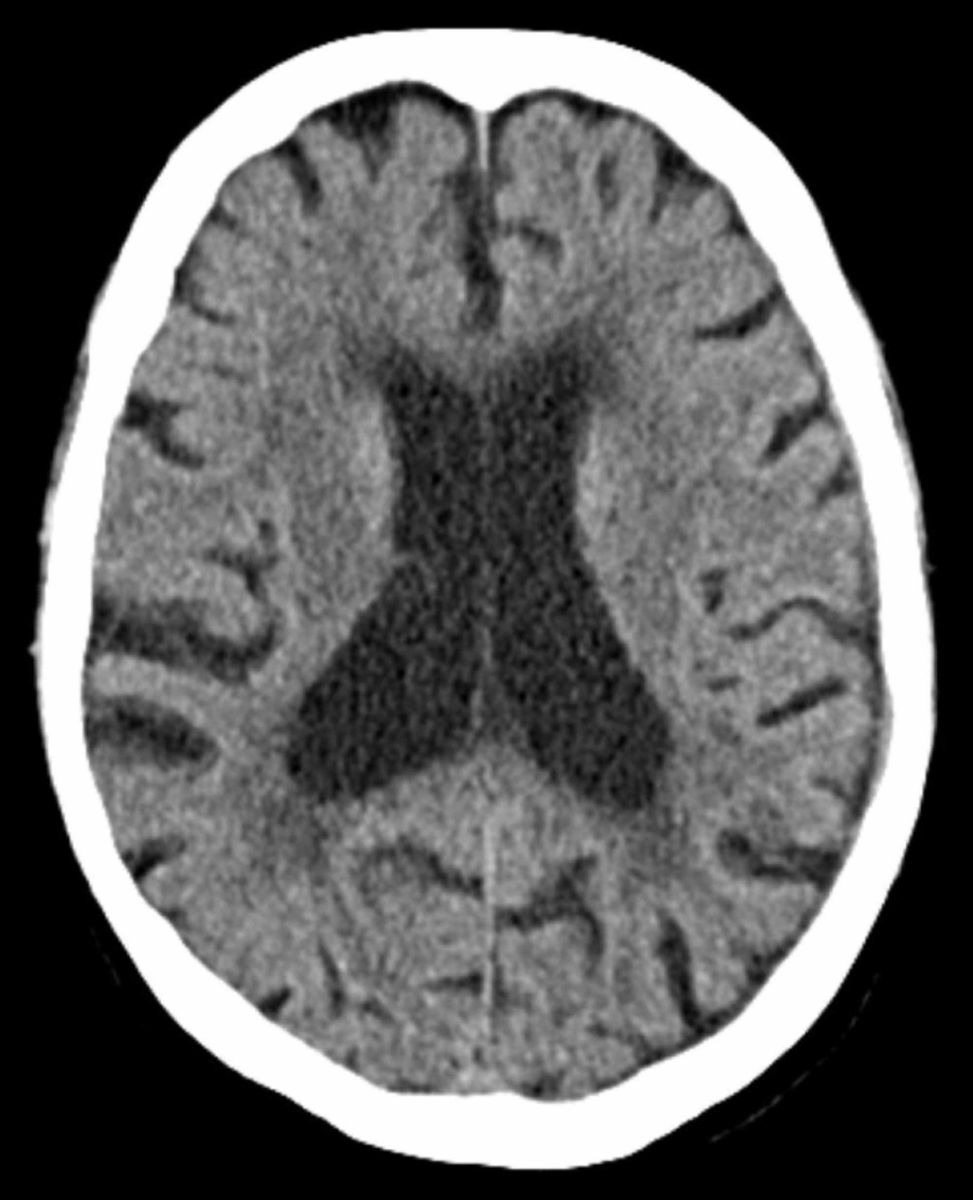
Ten-week outpatient follow-up CT examination demonstrating persistent thin subdural collection with minimal mass effect and no significant midline shift. CT: computed tomography
